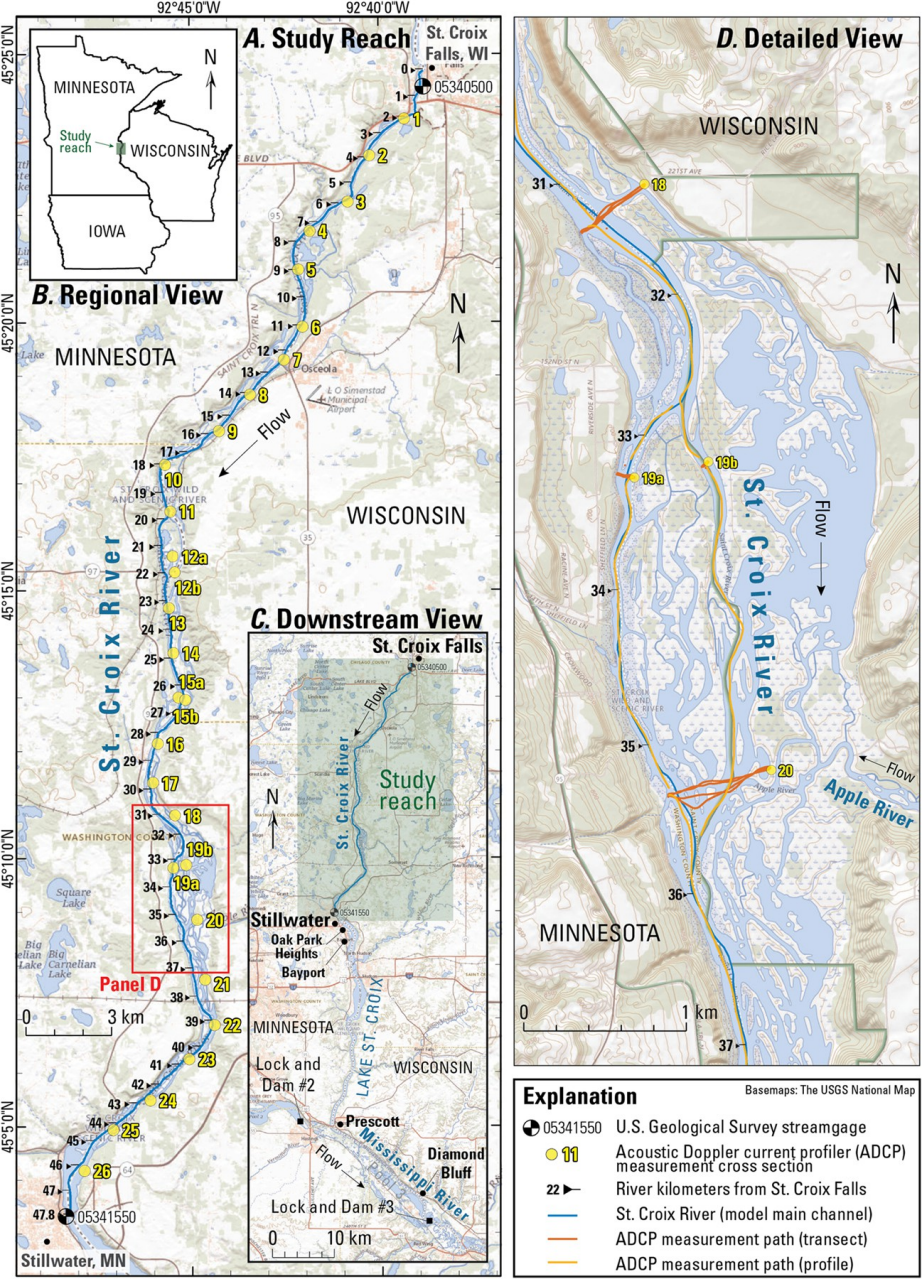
Study area overview map of the 47.8-kilometer St. Croix River study reach between St. Croix Falls, Wisconsin, and Stillwater, Minnesota. (A) acoustic Doppler current profiler (ADCP) data collected during June 19–22, 2018, where yellow dots show locations of transect stations; (B) regional overview inset map; (C) downstream map showing the study reach in the context of the lower St. Croix River to its confluence with the Mississippi River; (D) detailed inset image depicting ADCP data collection in a multi-thread segment of the study reach.
